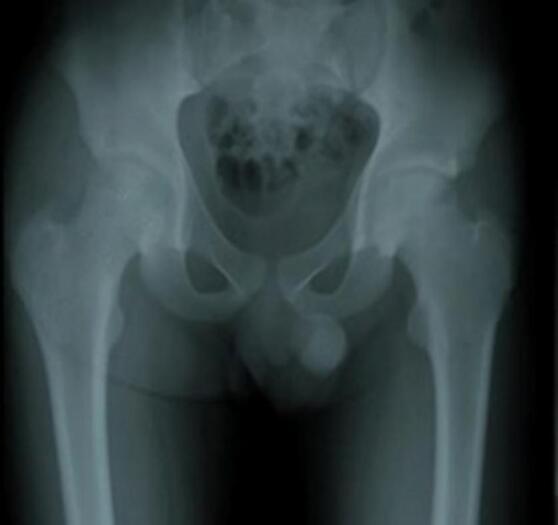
Postoperative Xray for patient I post core decompression.
